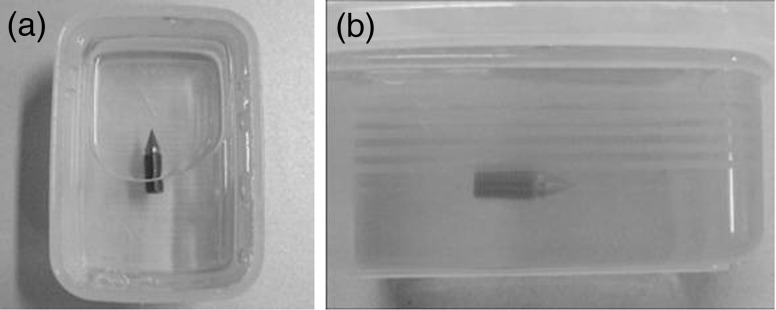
The quick fixation screw (QFS) was embedded in gelatin and its image was acquired using both 1.5-T and 3.0-T MR imaging scanners in order to visualize levels of susceptibility artifacts around the QFS. View from above (a) and lateral view (b).
